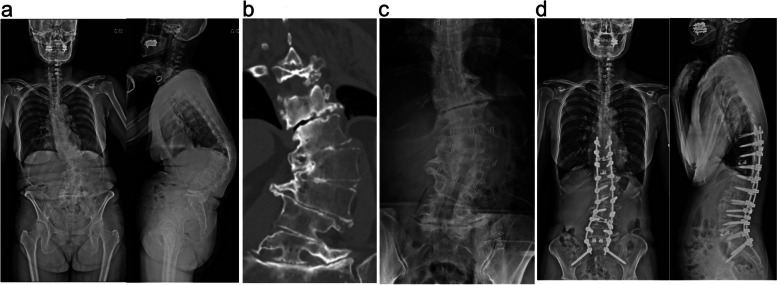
a Preoperative frontal and lateral view radiographs of a 62-year-old woman with low back pain demonstrated severe kyphosis and coronal spinal malalignment. b Multiple fused vertebrae at L1–2, L2–3, and L4–5 were found in multiplanar reconstruction of computed tomography. c The frontal radiograph exhibited LIR at L1/2 and L2/3 and XLIF at L3/4 were subseuqently performed. d Radiographs 1-year after surgery demonstrated that postoperative sagittal and coronal alignment were improved in this case. LIR; Lateral interbody release, XLIF; extreme lateral interbody fusion
